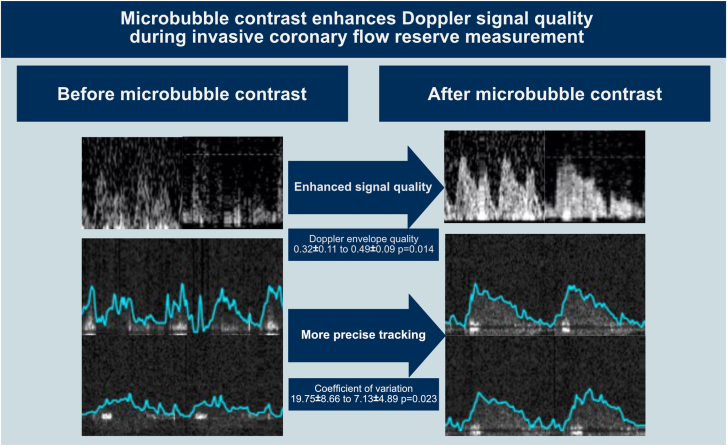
Microbubble contrast improves Doppler signals for coronary microvascular assessment. When the Doppler signal quality is poor, the administration of intravenous microbubble contrast improves the quality of the Doppler signal, and the console is able to track the flow velocity with greater accuracy. No significant improvement is seen when the Doppler quality is already good.
